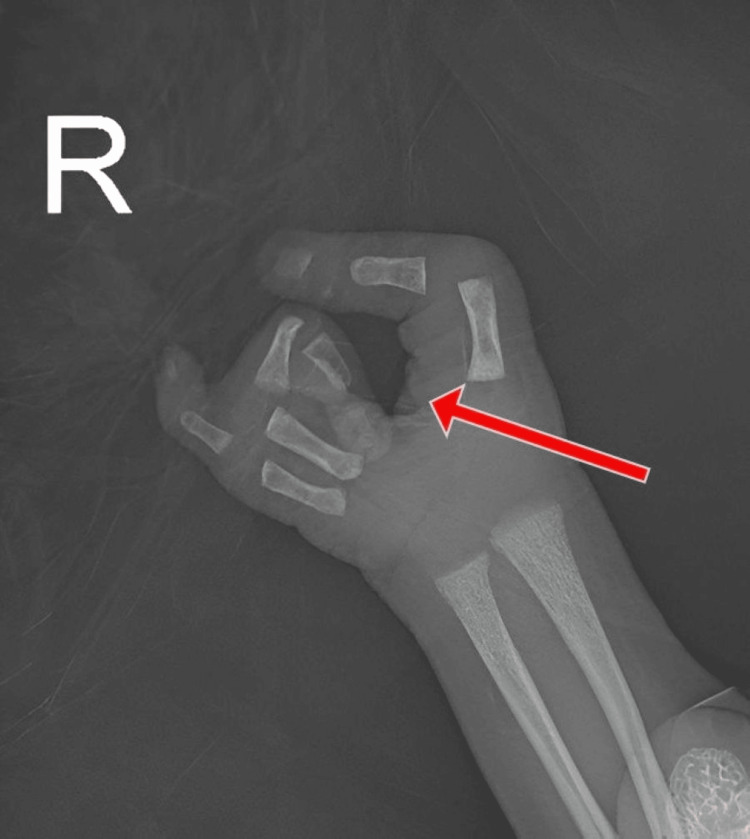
Plain radiograph of the right hand showing the absence of the index- and middle-finger phalanges and metacarpals
